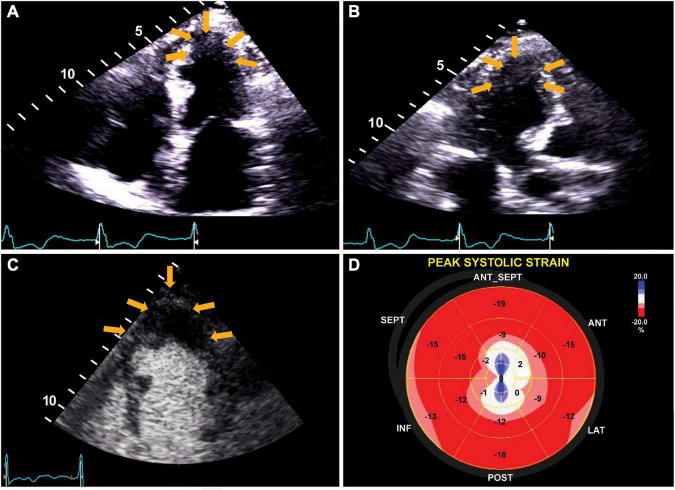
Transthoracic echocardiogram. The apical four-chamber view (A) (Supplementary Video 1), apical long-axis view (B) (Supplementary Video 2), and definity contrast echocardiogram (C) (Supplementary Video 3) show apical wall thickness (yellow arrows). Panel (D) shows strain analysis with markedly reduced peak systolic strain and evidence of dyskinesis in the apical segments.
